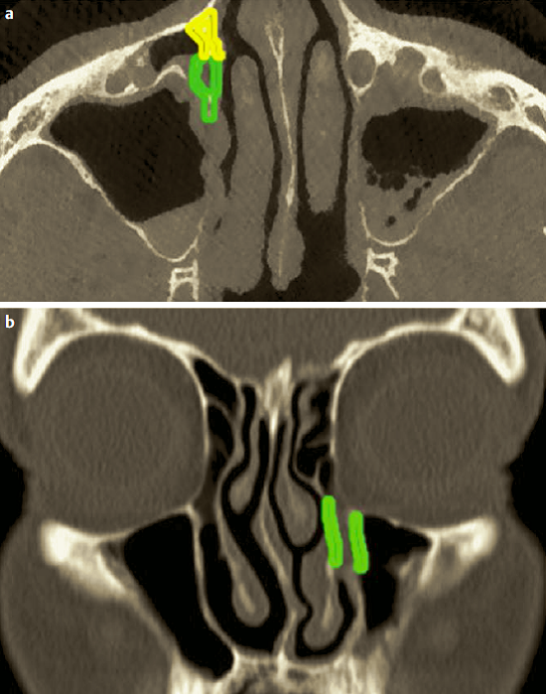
Extended maximal middle meatal antrostomy grade 4 (postlacrimal approach, [293]): Resection of the bone (green) medially, dorsally, and laterally of the nasolacrimal duct in order to mobilize it and to improve the insight into the maxillary sinus. Resection of the bone in case of prelacrimal access (yellow). a) axial CT scan, b) coronal CT scan.
